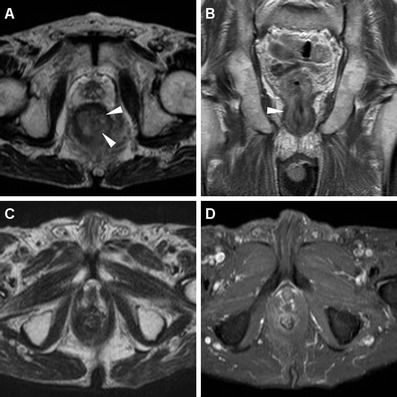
An 83-year-old man with known SCAC. Initial MRI shows moderate circumferential thickening of the anus with T2-hyperintense signal (a) and contrast enhancement (b) (*arrowheads*). Complete disappearance of the lesion is observed on axial T2 (c) and post-contrast fat-suppressed T1-weighted (d) images following chemo-radiotherapy
